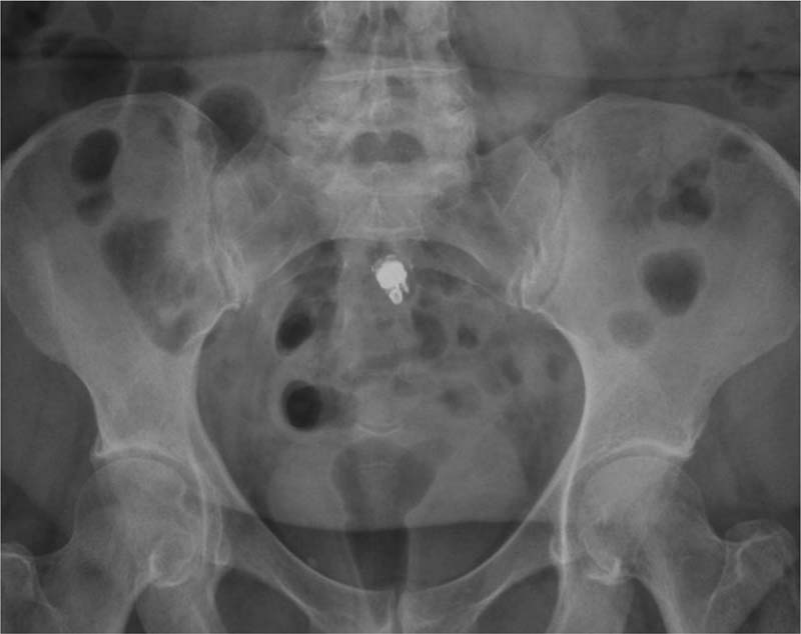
Radiographic appearance of retained capsule in terminal ileum.
